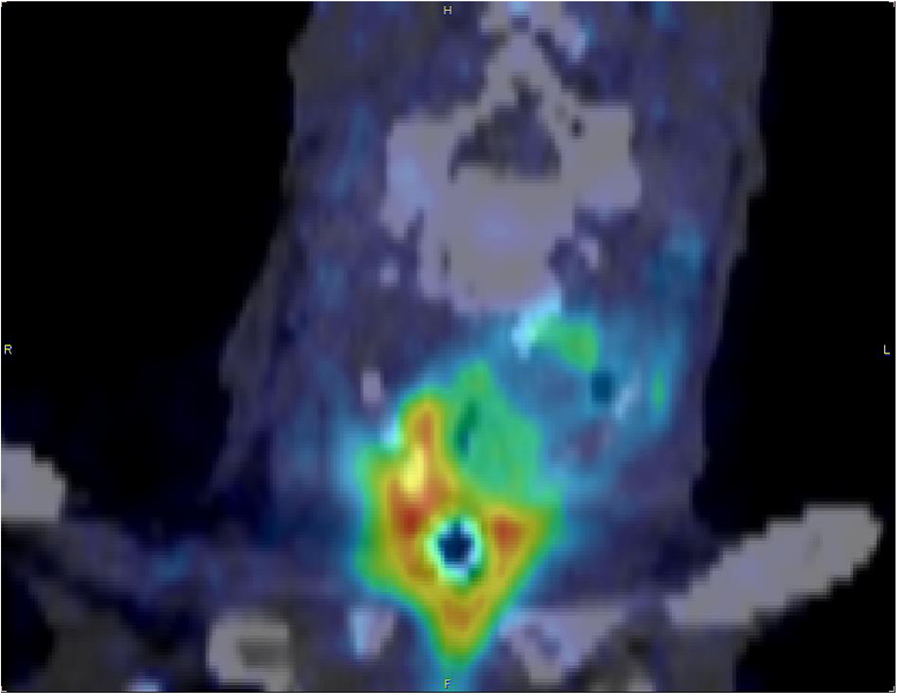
Fused PET and CT components of PET-CT scan. Coronal view of fused co-registered PET and ex vivo CT data for patient number 4. Very good alignment between the two data sets can be observed by using the tracheostomy as a reference point
